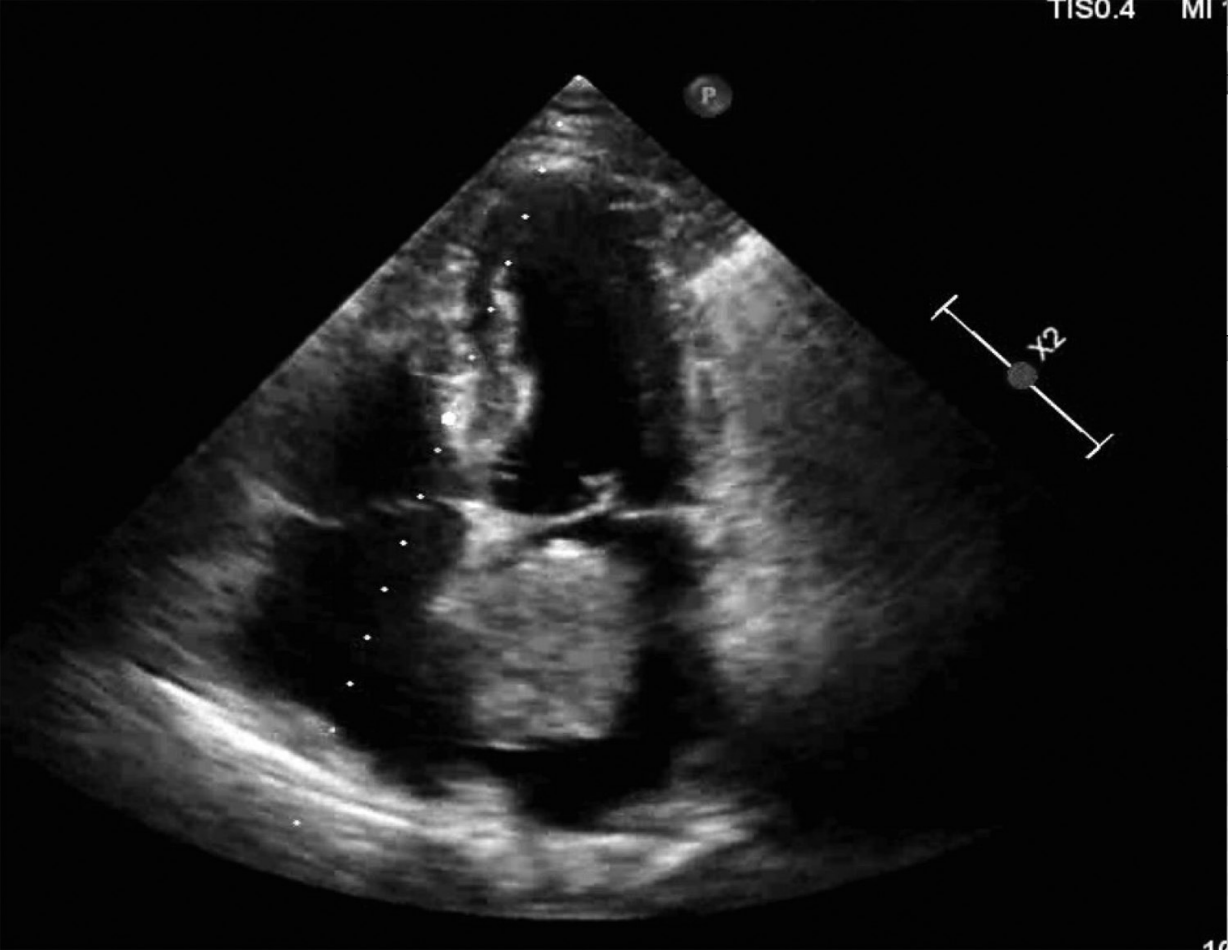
Echocardiography demonstration 48 mm left atrial mass attached to the fossa ovalis of the /atrial septum typical for cardiac myxoma.
